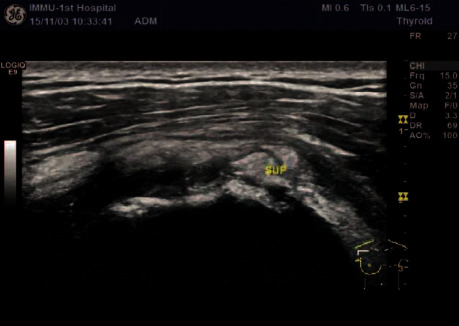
Two-dimensional ultrasound supraspinatus tendon injury.
